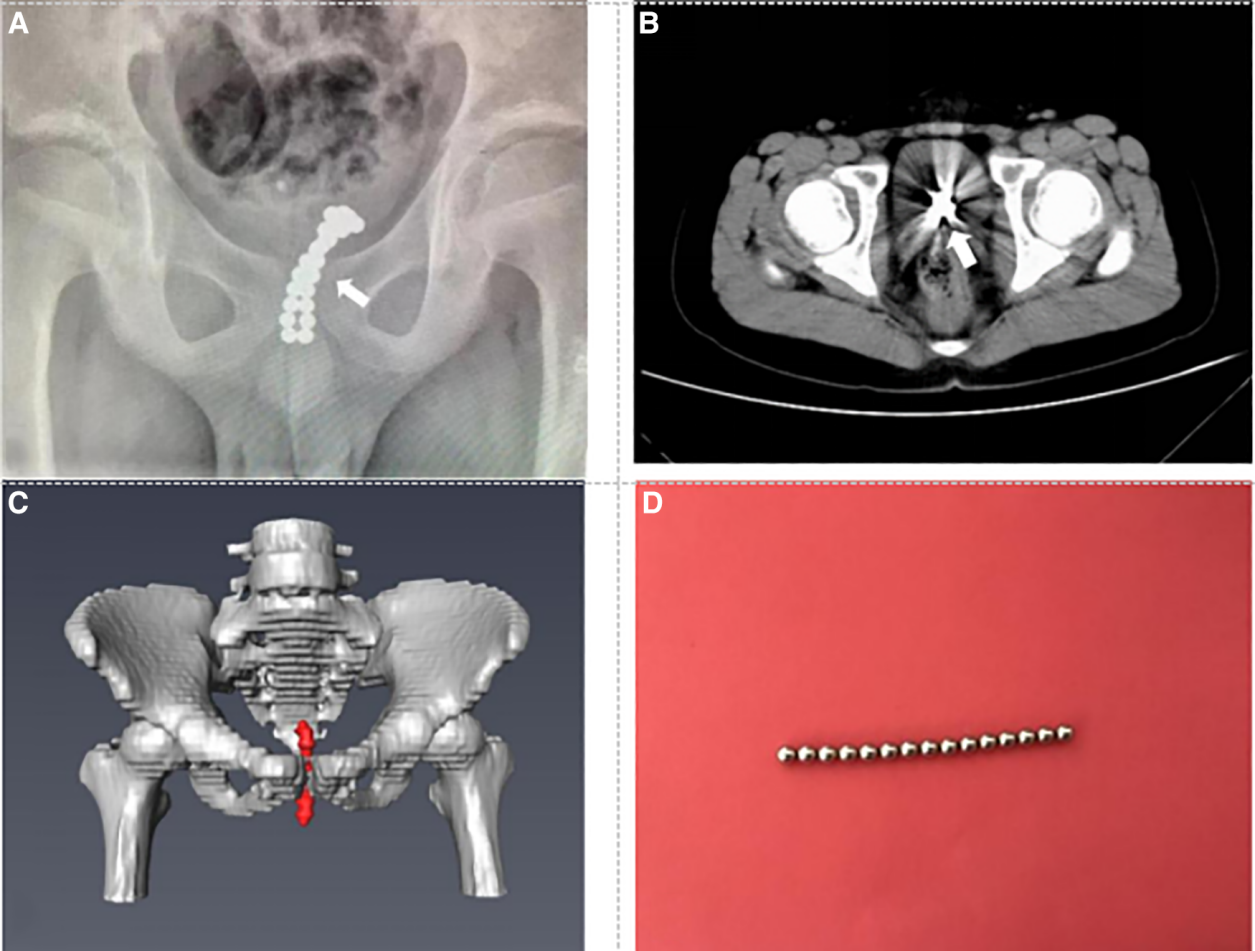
Preoperative imaging data and foreign body. (A) Plain film x-ray shows multiple small spherical foreign bodies in the bladder (see arrow). (B) CT of the pelvis shows the massive foreign body in the bladder (see arrow). (C) Three-dimensional reconstruction CT shows the stripe foreign body in the bladder (red mark). (D) Foreign bodies are multiple magnetic beads (partly).
